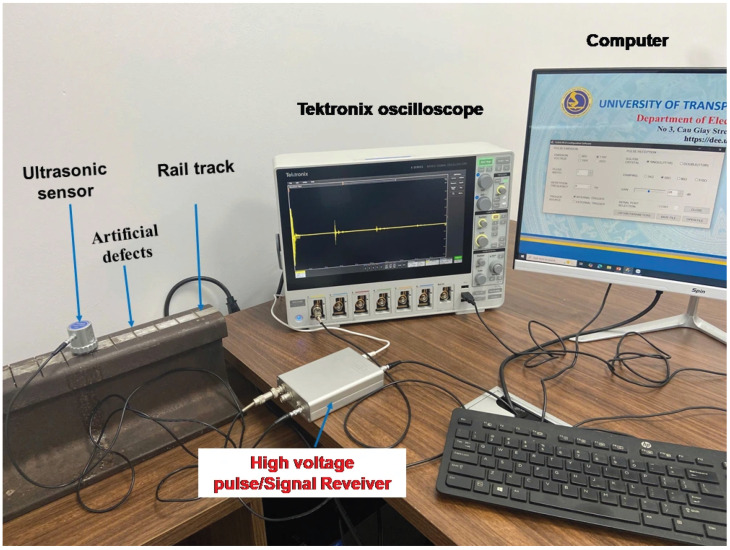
Photo of completed ultrasonic non-destructive testing system for rail track inspection.
